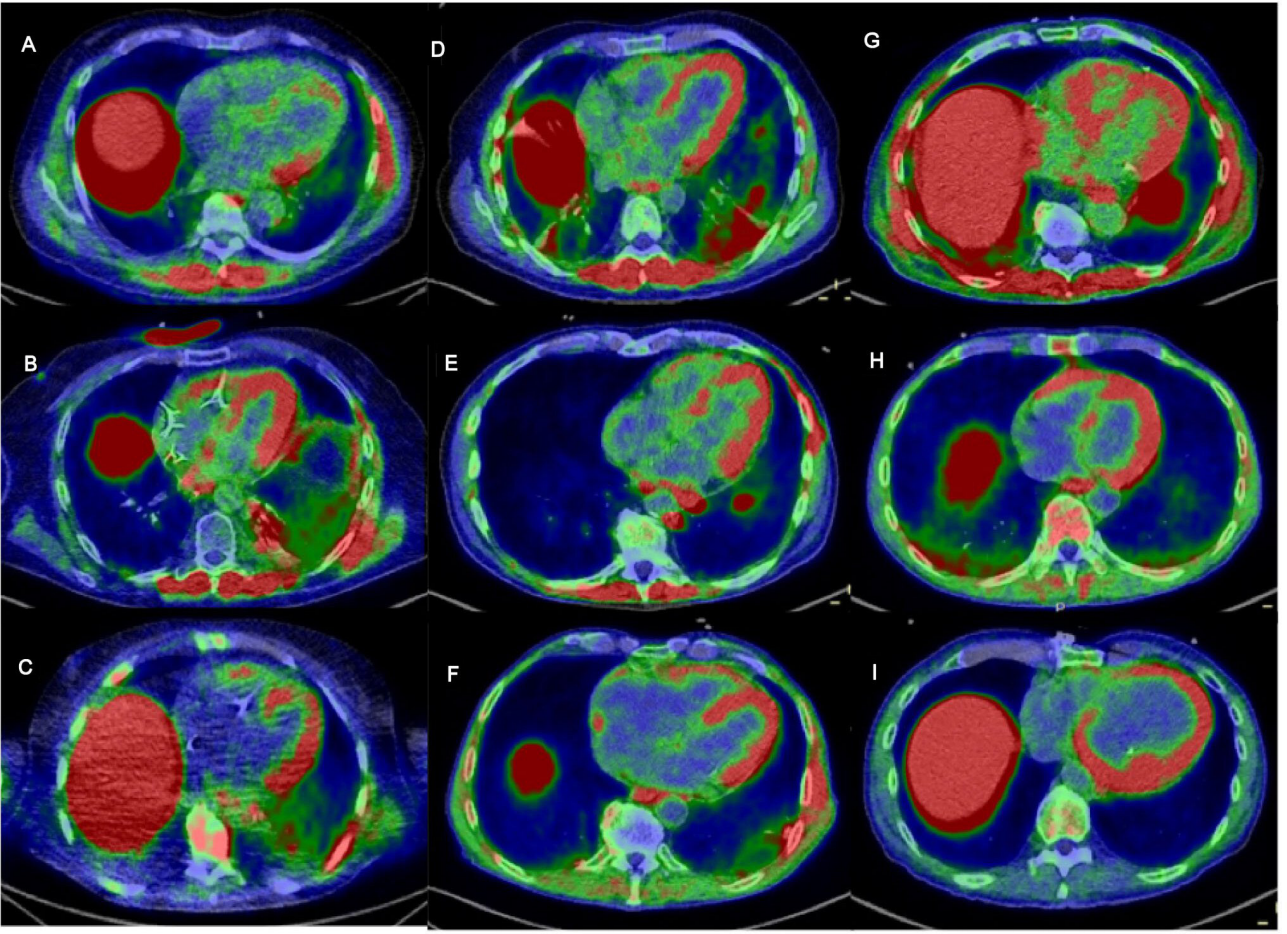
Fused ^68^Ga -DOTATOC PET/CT images in the axial plane centered on the long axis of the LV (A–I): patients 3–11 with pathological uptake in the myocardium; pathological uptake in the paravertebral and intercostal muscle is also seen in some patients (A, B, D, E, G); SUV scale 0–2 g/mL. ^68^Ga -DOTATOC, ^68^Ga-DOTA(0)-Phe(1)-Tyr(3)-octreotide; LV, left ventricle.
